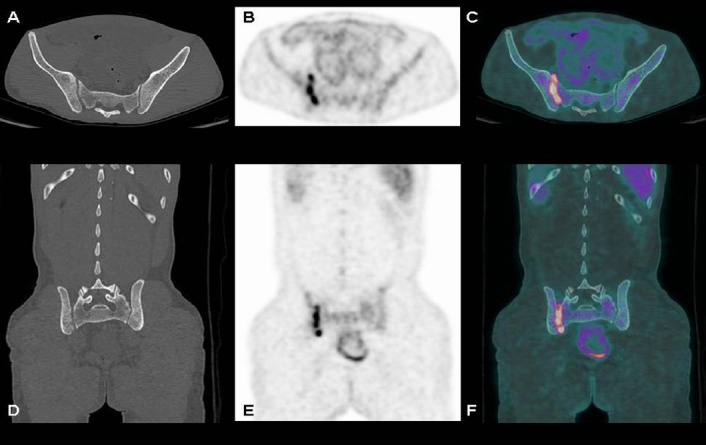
Anterior axial CT (A), PET (B), and fused PET/CT (C) images, coronal CT (D), PET (D) and fused PET/CT (E) images documenting increased tracer uptake at the right sacroiliac joint (SUV_max_=8.9) in absence of bone alteration, suggesting the presence of a sacroiliitis.
